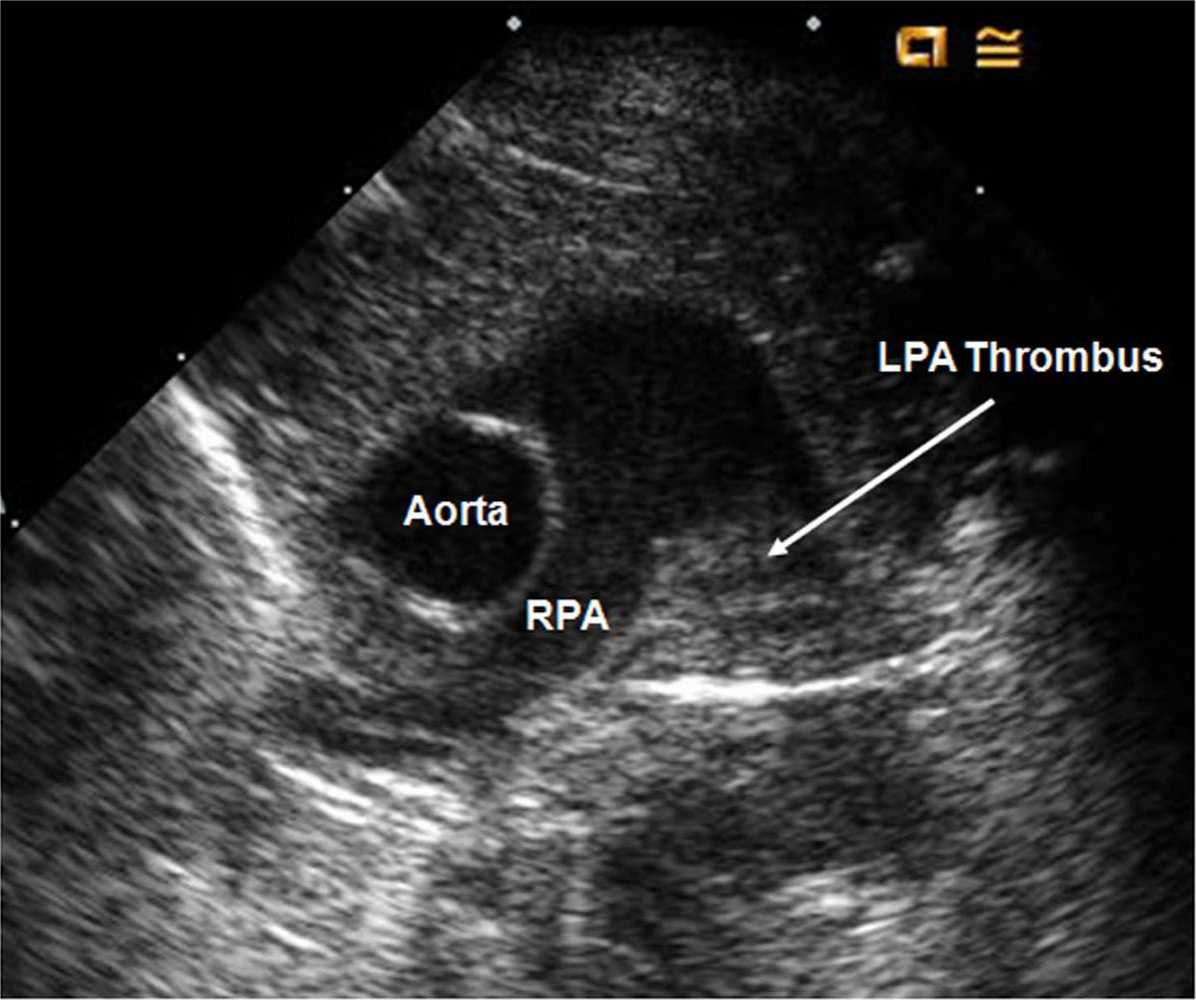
Cross-sectional view on echocardiogram suggesting the presence of a thrombus in the left pulmonary artery.
